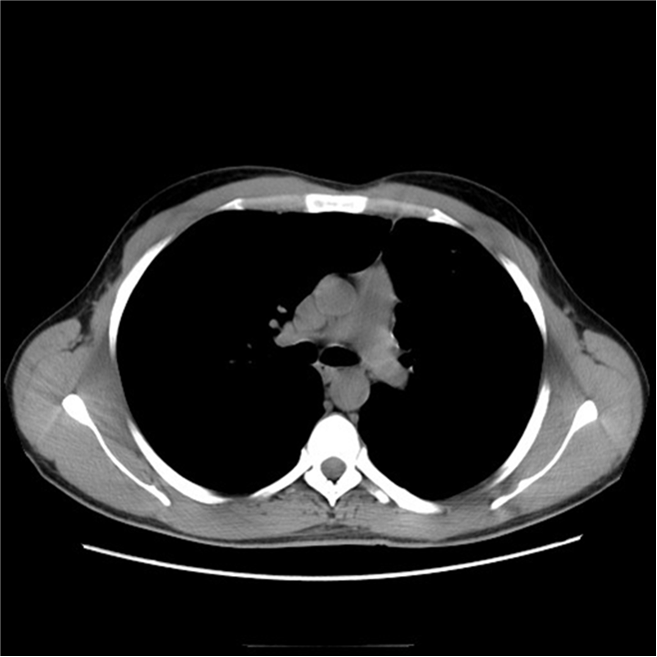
Computed tomography scan of the chest: there is no evidence of disease 5 months after treatment.
